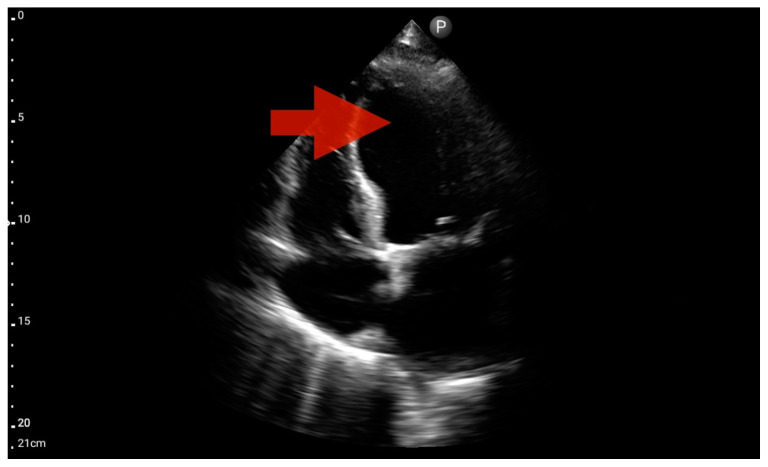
Left ventricle hypokinesis; position: apical four-chamber (FATE protocol). Sector probe; the red arrow marks the left ventricle, which, during the examination, showed signs of reduced ejection fraction and hypokinesis (source: author’s material—DK).
